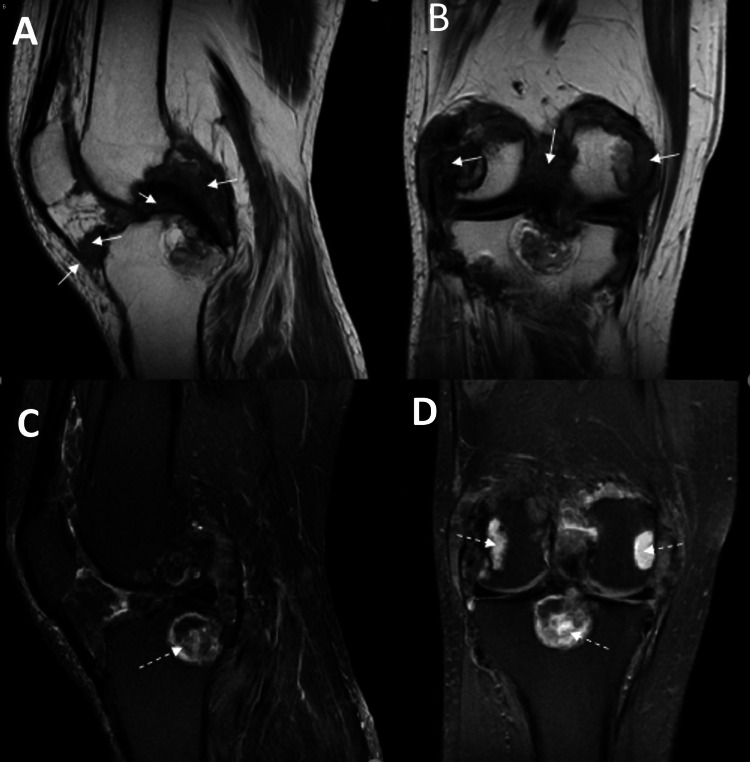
MRI of the right knee T2-weighted sagittal view (A), coronal view (B). T1-weighted sagittal view (C), coronal view (D). The arrows point to the location of the intra-articular tophi MRI: magnetic resonance imaging
